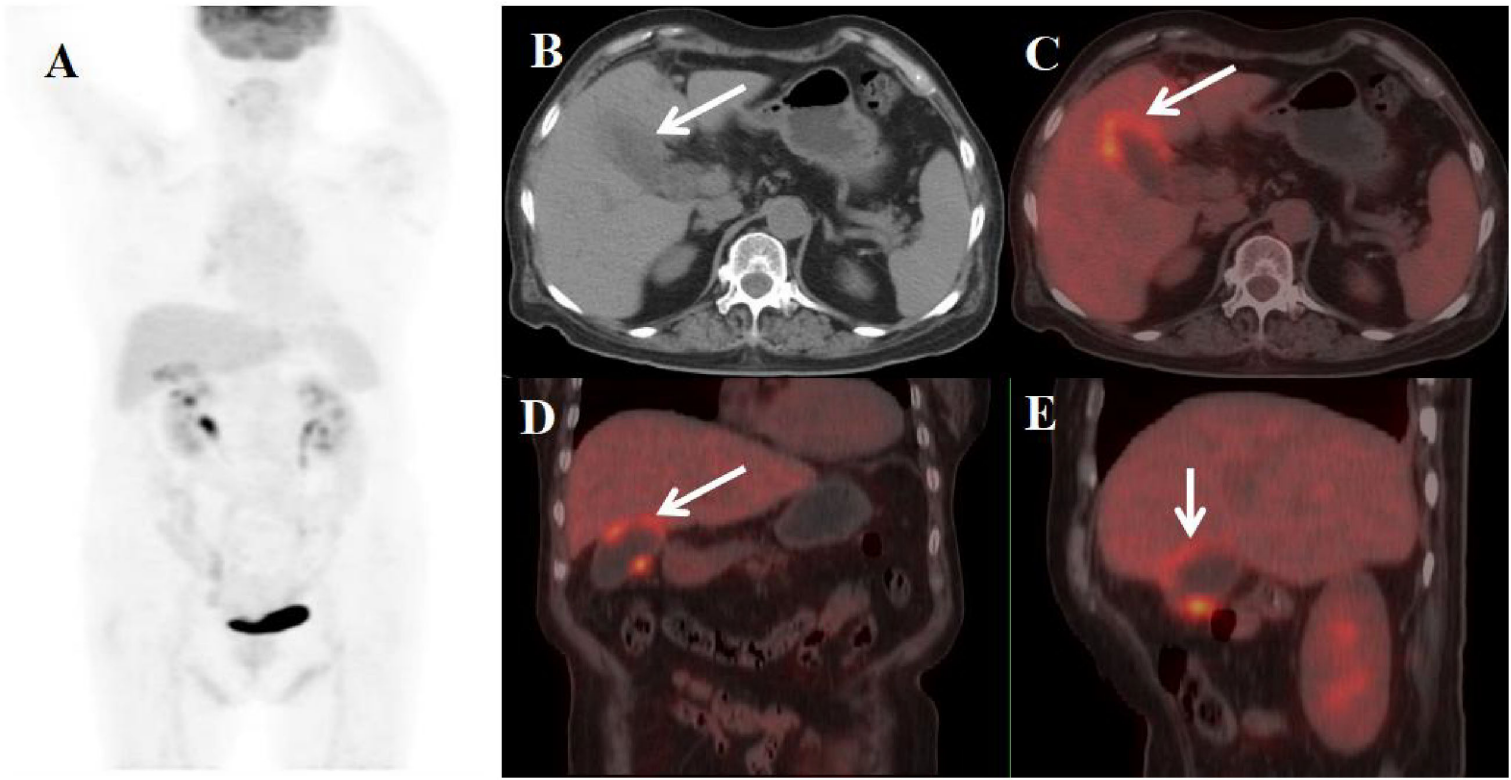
Female, 70 years old. (A) (whole body MIP), (B, C) (Axial CT and fused axial PET-CT), (D) (Fused coronal PET-CT), and (E) (Fused sagittal PET-CT). 18F-FDG PET/CT Imaging Findings: The gallbladder demonstrates focal mural thickening (maximum 12 mm) with overall enlargement (B, C, arrow ↑), while maintaining mucosal integrity and a distinct hepatobiliary interface. These morphological changes are accompanied by localized FDG avidity (SUVmax 5.8) (D, E, arrow ↑).
